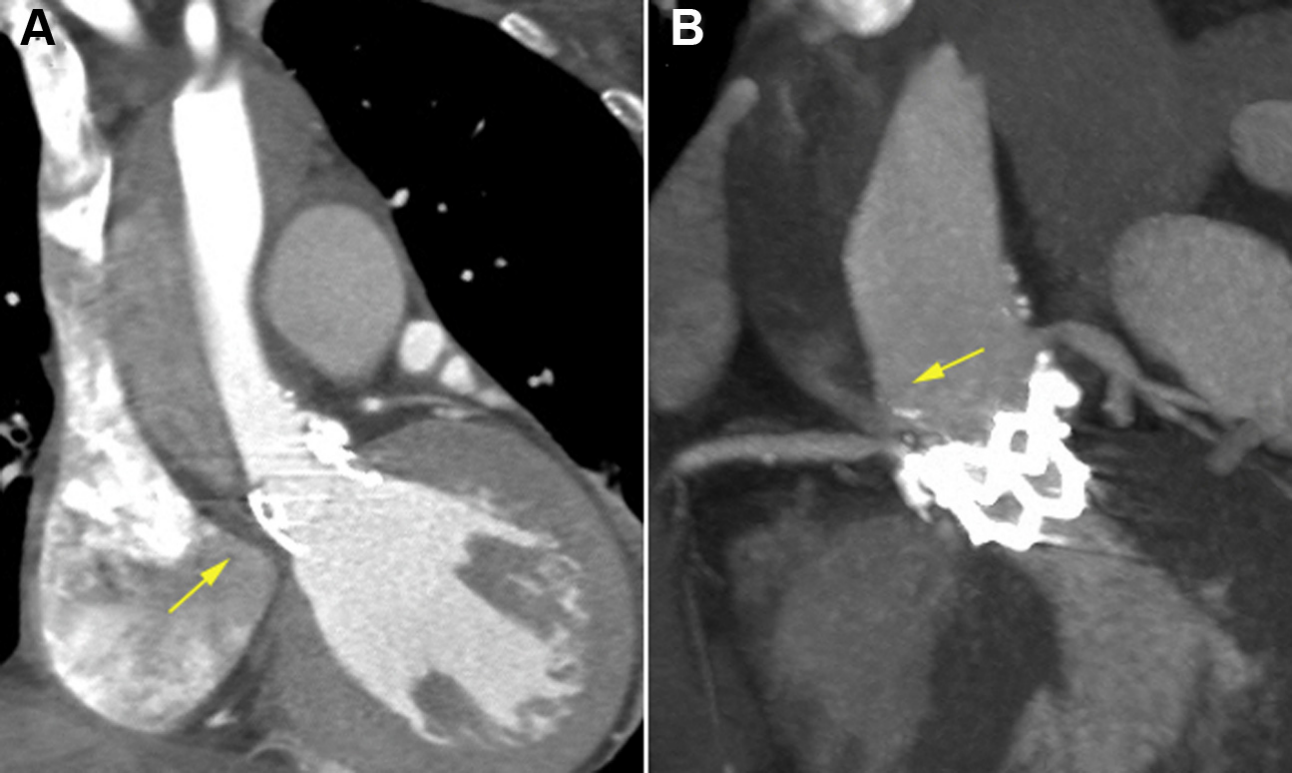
Computed Tomography Images Showing the Site of Intimal Tear Postoperative day 2 computed tomography images reveal the dissection flap originating from the aortic wall covered with the stent frame (A) and propagating spirally through the aortic wall above the right coronary artery ostium (B).
